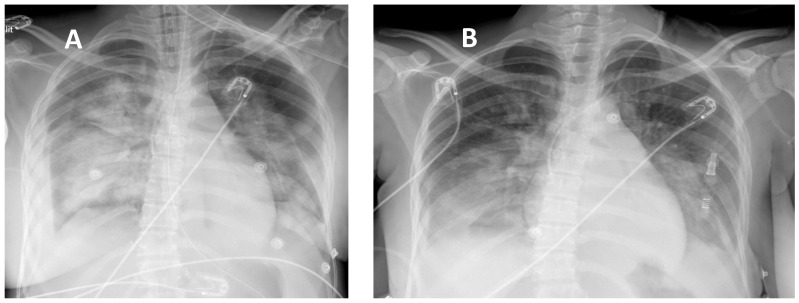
(A) Chest X-rays showing persistent lung edema with bilateral infiltrates under mechanical ventilation and extracorporeal membrane oxygenation; (B) chest X-rays showing partial regression of lung edema and improved aeration on spontaneous ventilation after tracheal extubation.
